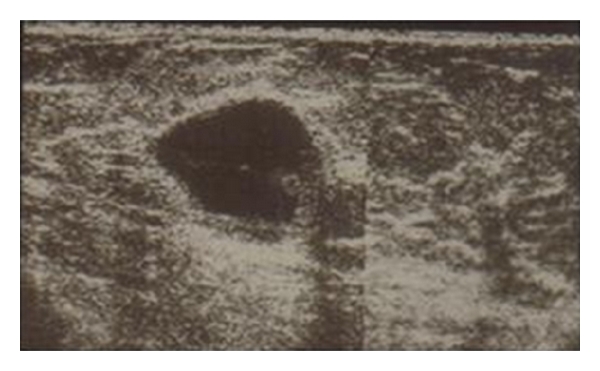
Ultrasonography showed a cystic mass of 27 mm, with posterior acoustic enhancement, and a solid component.
